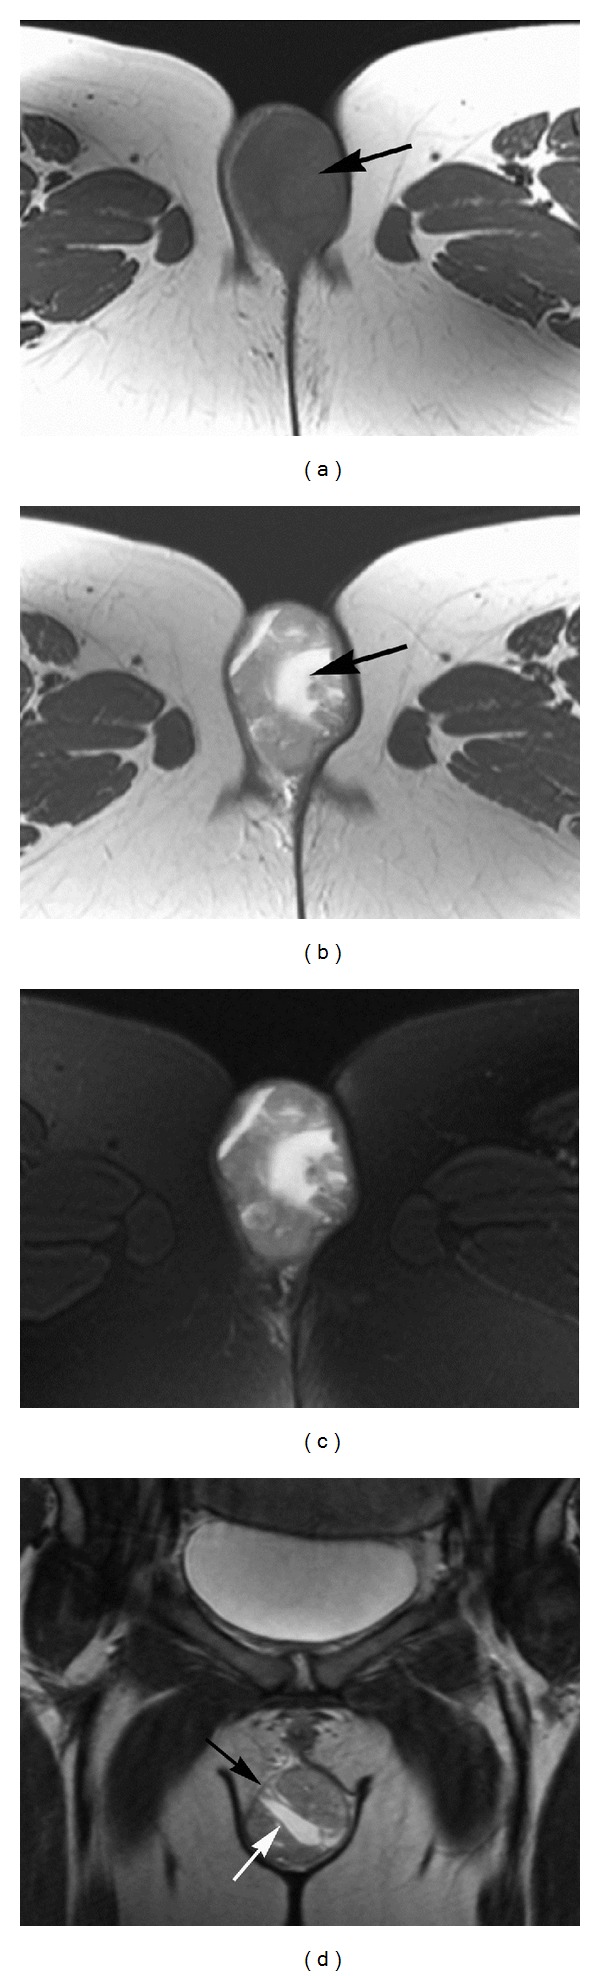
Magnetic resonance images of the pelvis. (a) Axial T1-weighted spin-echo image (TR = 700 ms/TE = 10 ms). A well-defined, ovoid mass in the subcutaneous tissue of the inferior right labia majora is seen (arrow), with smooth contours and no evidence of infiltration of adjacent structures. The mass has homogenous signal which is isointense to skeletal muscle. (b) Axial T2-weighted fast spin-echo image (TR = 4450 ms/TE = 89 ms). The mass has heterogeneous high signal intensity, with areas of very high signal intensity suggesting the presence of fluid (arrow) (c) Axial T2-weighted fast spin-echo image with frequency selective fat saturation (TR = 5266 ms/TE = 89 ms). No inflammatory changes are seen in the adjacent fat. (d) Coronal T2-weighted fast spin-echo image (TR = 5216 ms/TE = 83 ms). A well-defined thin capsule around the mass (black arrow) and thin internal septa (white arrow) are demonstrated.
